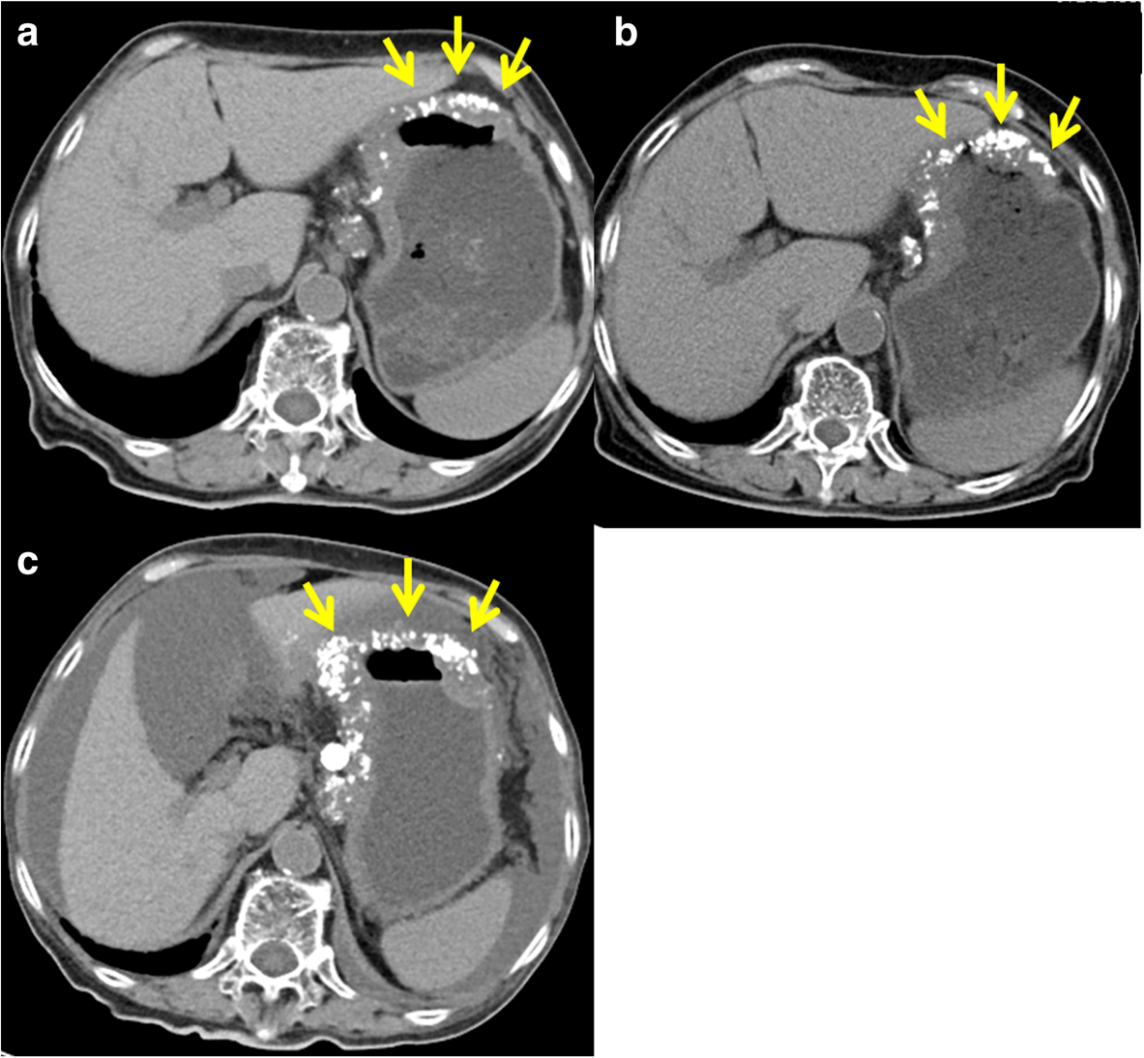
Contrast-enhanced computed tomography images of mucinous gastric carcinoma. There is diffuse low attenuation in the thickened gastric wall along with punctuate calcifications (*yellow arrows*), which increase in number with successive images. a Prior to chemotherapy. b After three cycles of chemotherapy. c One month after chemotherapy ends. Massive ascites has accumulated within the peritoneal cavity
